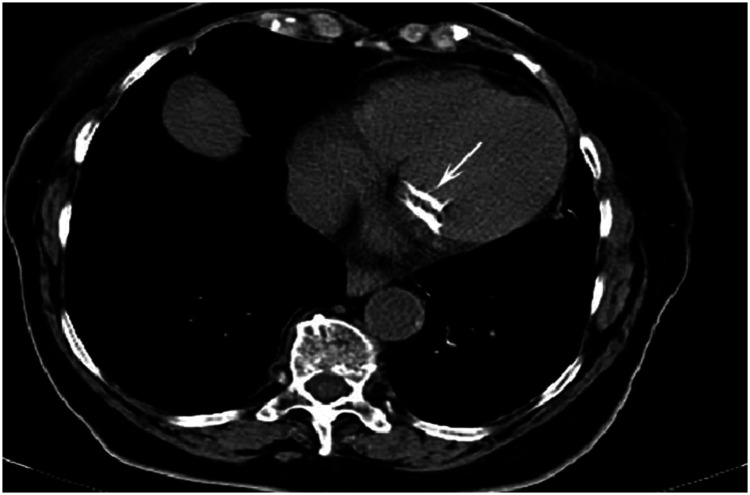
Axial cardiac computed tomography image shows the presence of annular mitral calcification (white arrow) (used with permission).
